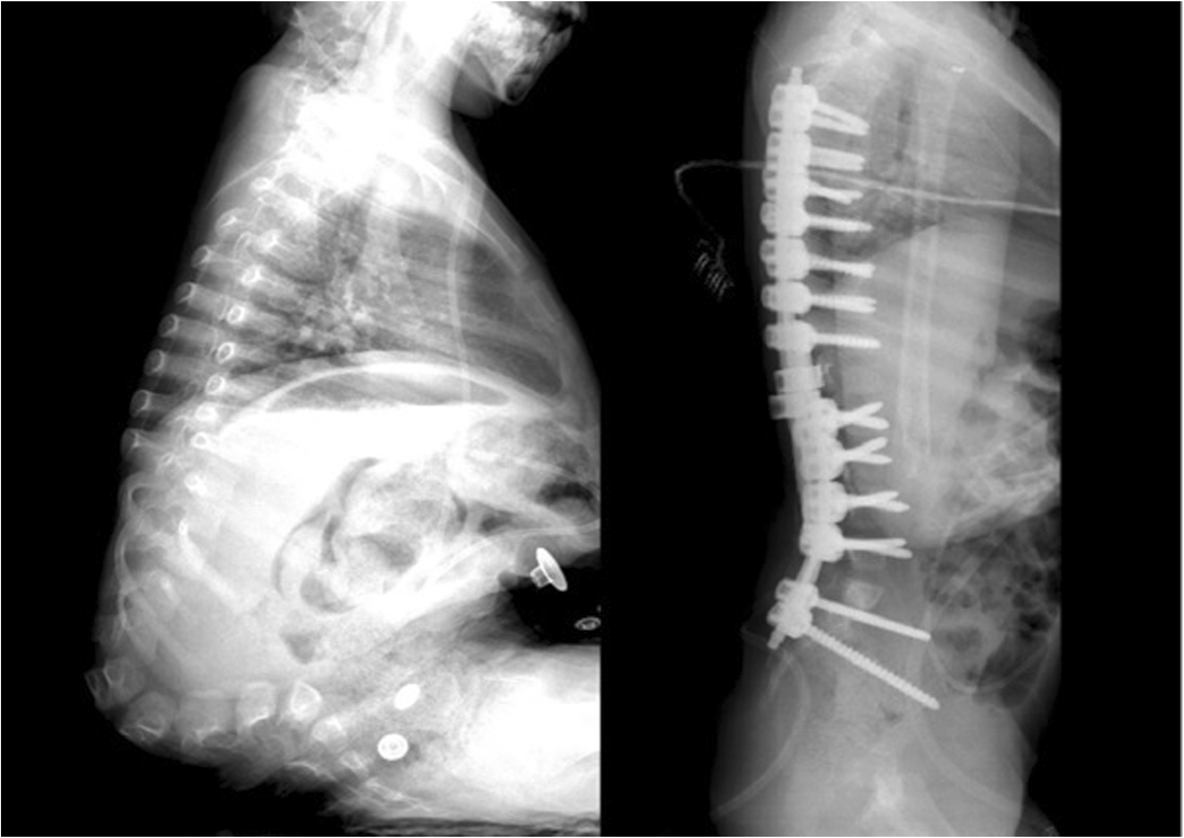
A 5-year-old male patient. Pre- and early postoperative lateral radiography
